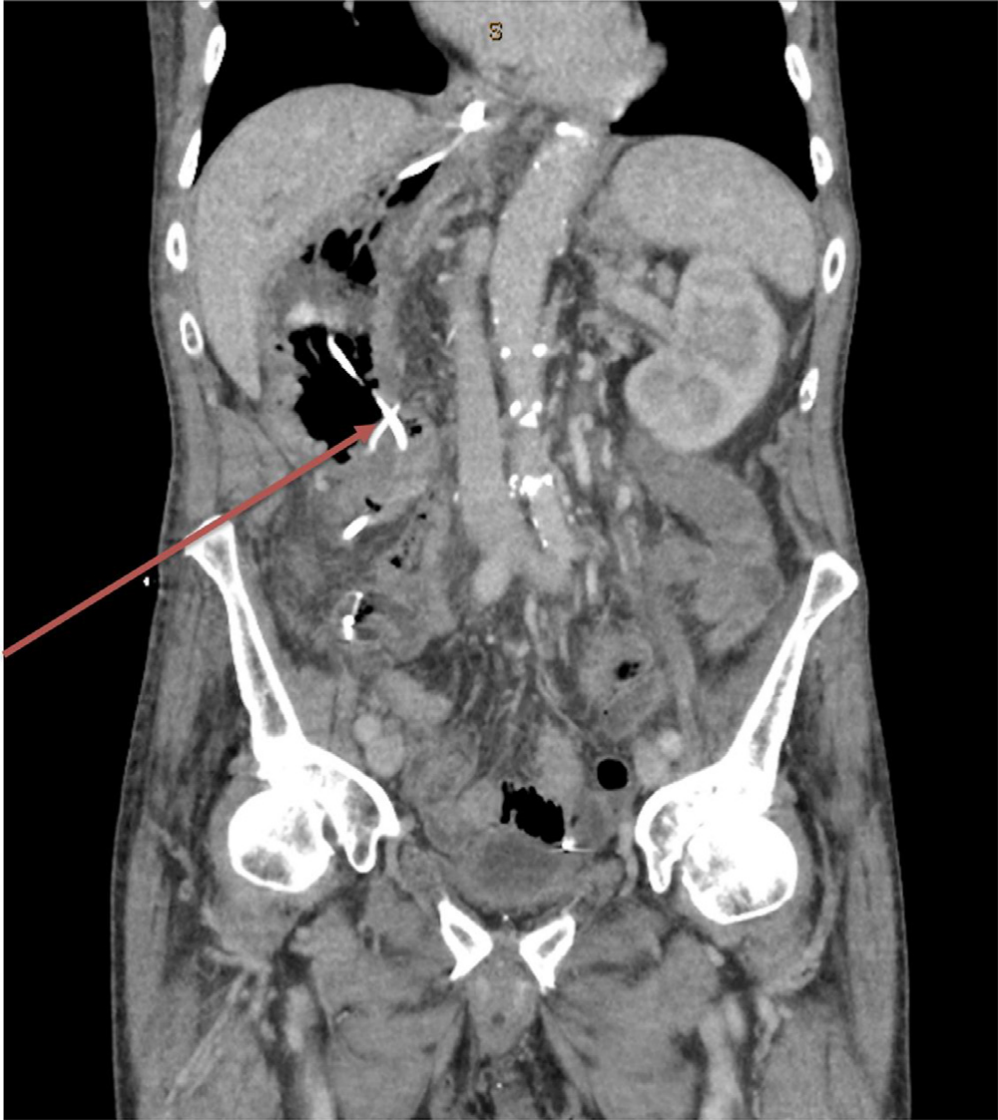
Coronal slice of CT chest, abdomen and pelvis with contrast post gastrojejunostomy, demonstrating a decompressed right-sided stomach. Red arrow – Decompressed right-sided stomach with nasojejunal tube bypassing the anastomosis.
